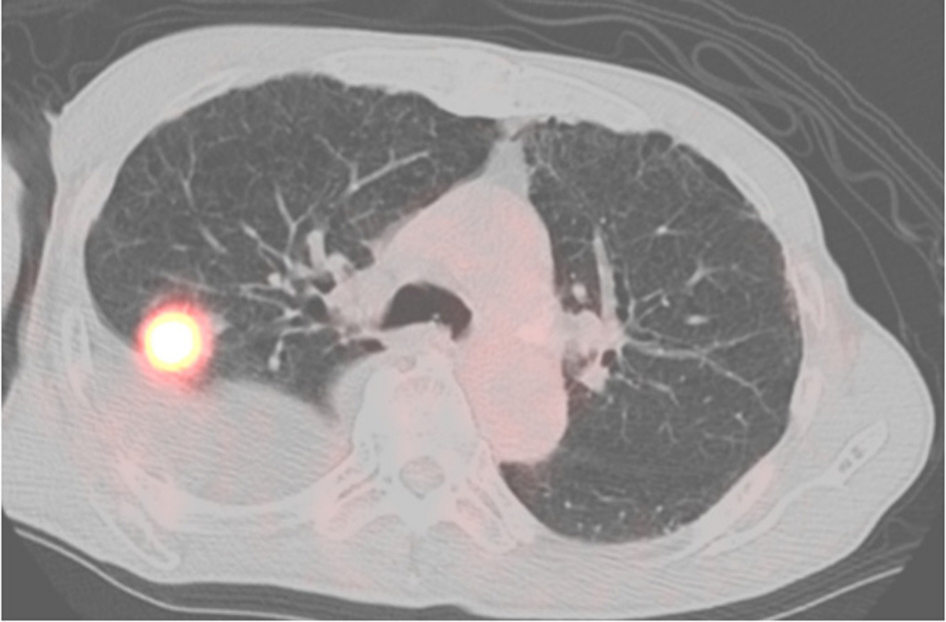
PET-CT scan showing no FDG uptake in the pleura.
